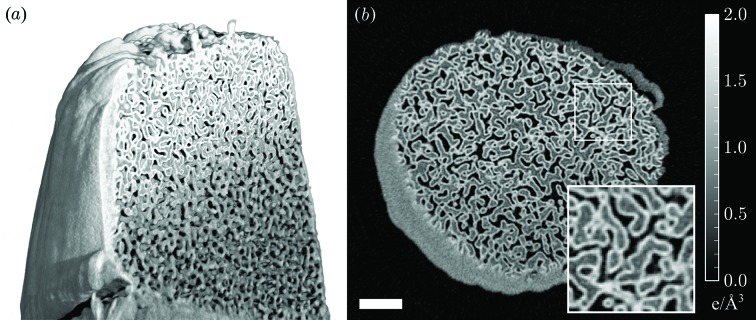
X-ray ptychographic tomography of a nanoporous glass sample. (*a*) Rendering of three-dimensional reconstruction with 22 nm resolution shows a gradient of the thickness of the Ta_2_O_5_ conformal coating in the axial direction. (*b*) Axial section with a clear differentiation between air, glass and the conformal coating. The scale bar is 1 µm. The inset in (*b*) corresponds to the 1.5 µm × 1.5 µm region indicated with a white rectangle.
